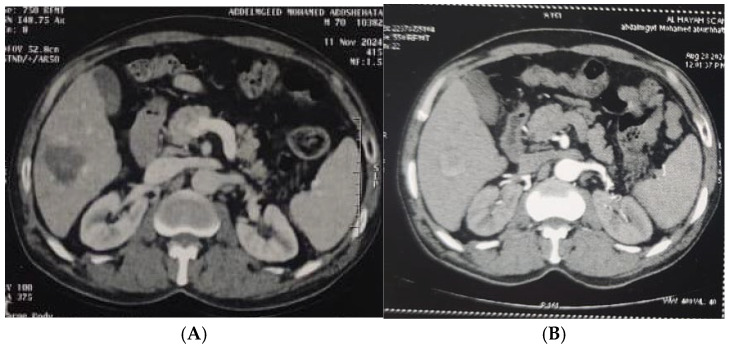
(A). Triphasic CT abdomen (arterial phase) axial cuts revealing right lobe segment VI focal lesion with homogeneous enhancement (before MWA). (B). The same lesion (arterial phase) axial cuts one month after MWA.
